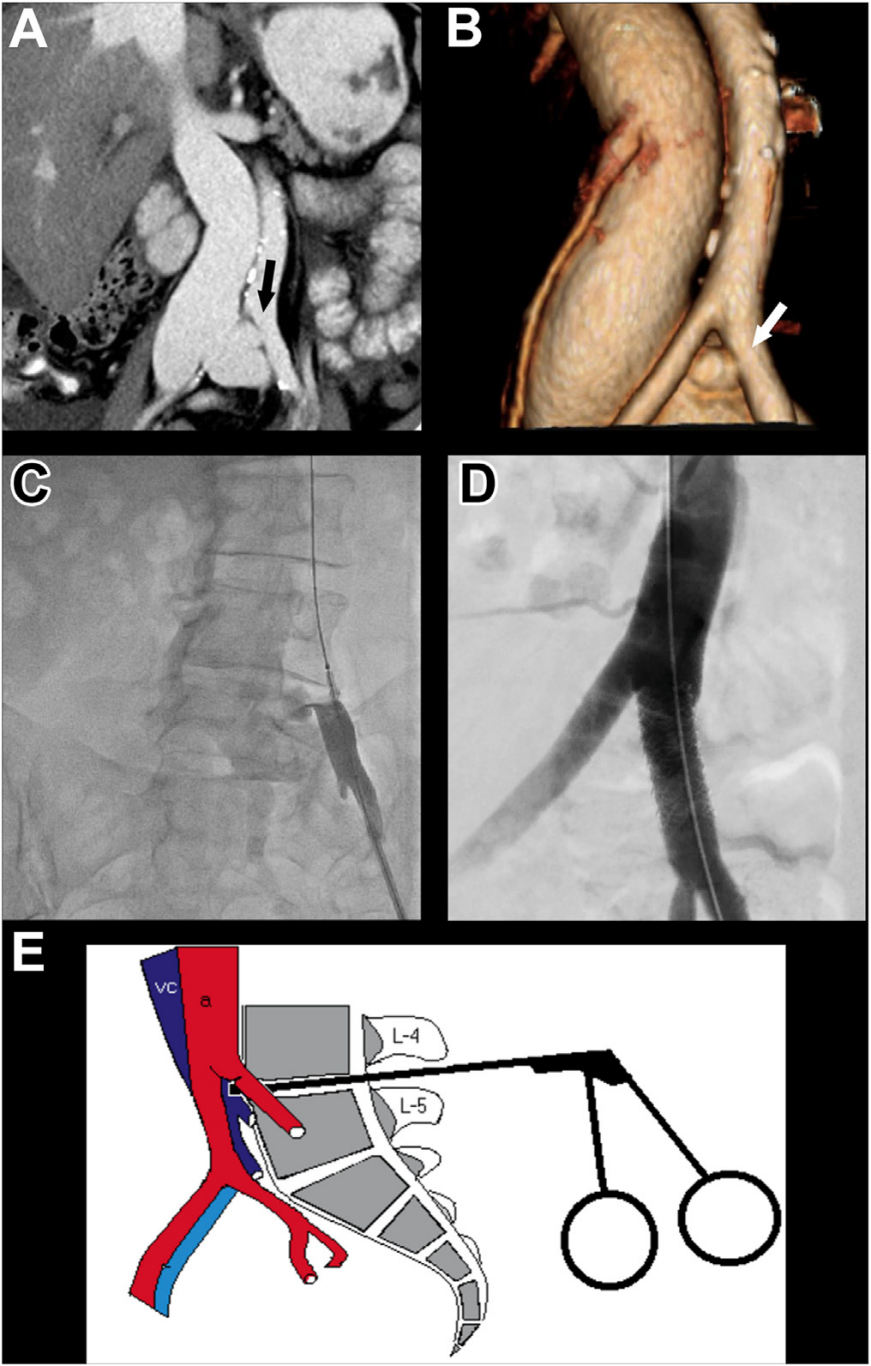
Iliac arteriovenous fistula—diagnosis and management. (A) Computed tomography angiography (CTA) showing enlargement of the inferior vena cava compared with the aorta and an arteriovenous (AV) fistula between the left common iliac artery and the ipsilateral vein (arrow). (B) 3D reconstruction of the CTA scan shown in (A) and the AV fistula (arrow). (C) Aortography identifying the fistula and a large contrast shunt between the left common iliac artery and vein. (D) Aortography after closure of the AV fistula with a 9 × 32 mm Advanta covered stent. (E) Anatomy of the iliac vessels in relation to the L4-L5 intervertebral disc and laminectomy. From Papadoulas et al,^1^ with permission of the author.
